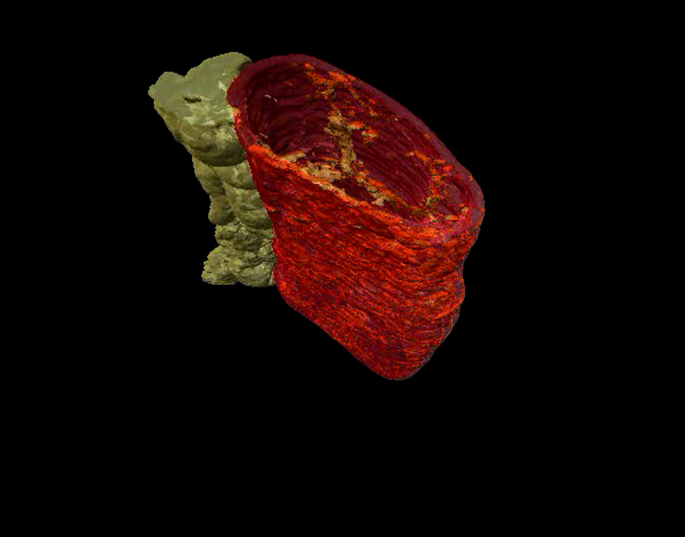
This movie shows high-resolution magnetic resonance (MRI) images of cells labeled with micron sized iron oxide particles seeded onto a natural small intestine scaffold. The movie starts transversing through short axis MRI images along the major axis of the intestine. A few seconds after the start, the volume rendering of segmented cells (brown, threshold of hypointensities), the intestine membrane (red) and the mesentery (yellow) is activated. (MPEG; 27 MB).
